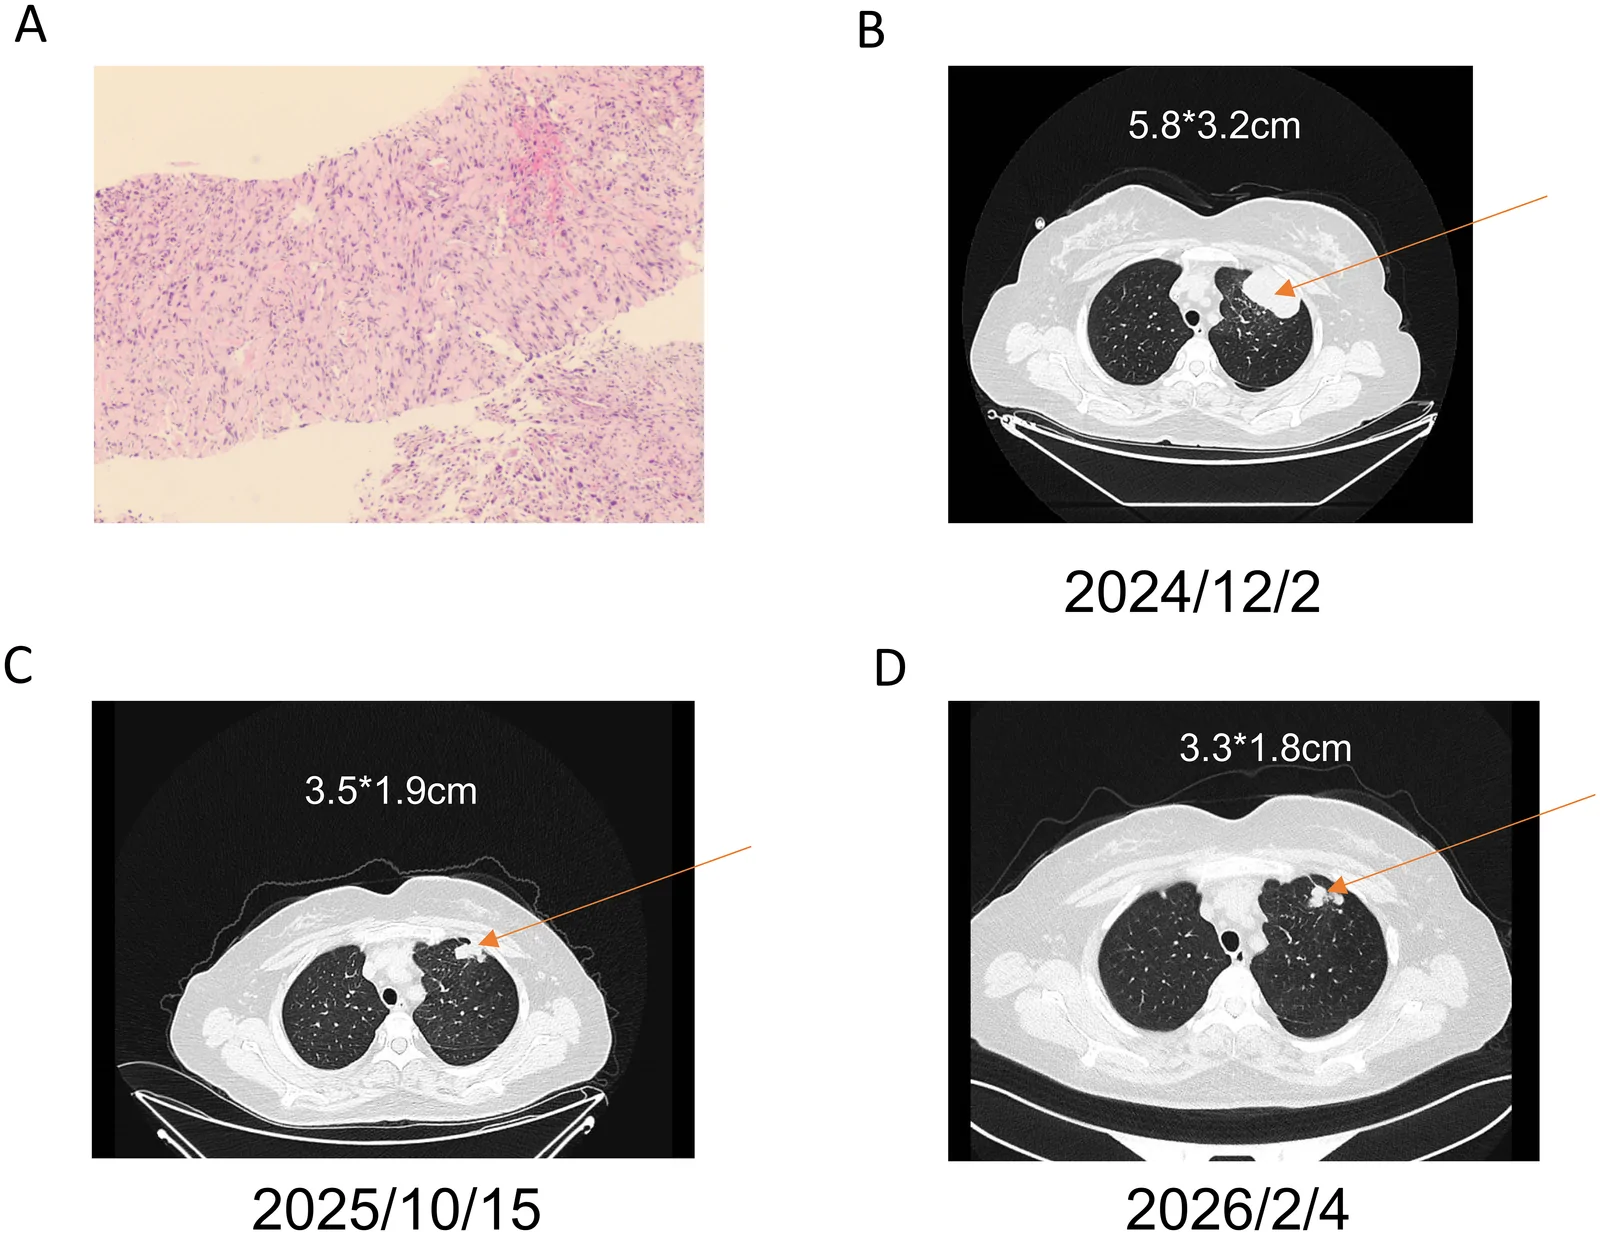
Representative case of PD-1 inhibitor combined with chemotherapy for metastatic undifferentiated pleomorphic sarcoma. Representative images. (A): Needle biopsy of the left lung mass confirmed a pathological diagnosis of metastatic undifferentiated pleomorphic sarcoma. (B): Chest CT before treatment (December 2, 2024) showing a left lung metastasis measuring approximately 5.8 × 3.2 cm. (C): Serial CT scans during treatment (October 15, 2025) showing progressive shrinkage of the left lung metastasis, measuring 3.5 × 1.9 cm. (D): Post-treatment CT (February 4, 2026) showing further reduction of the left lung metastasis to 3.3 × 1.8 cm, with best response confirmed as partial response (PR).
